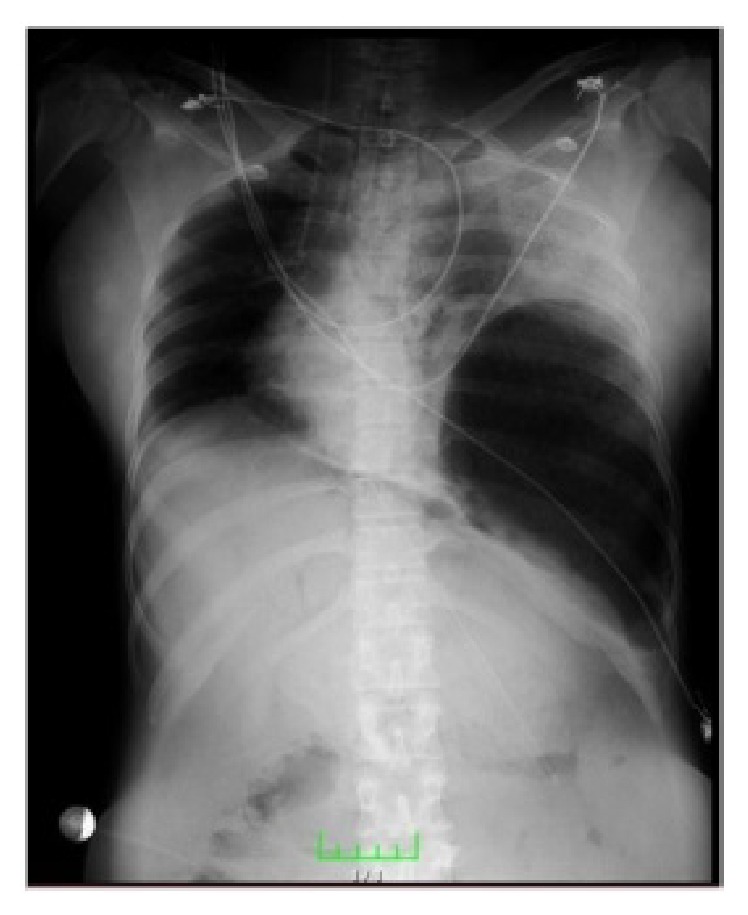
X-ray subdiaphragmatic free gas.
